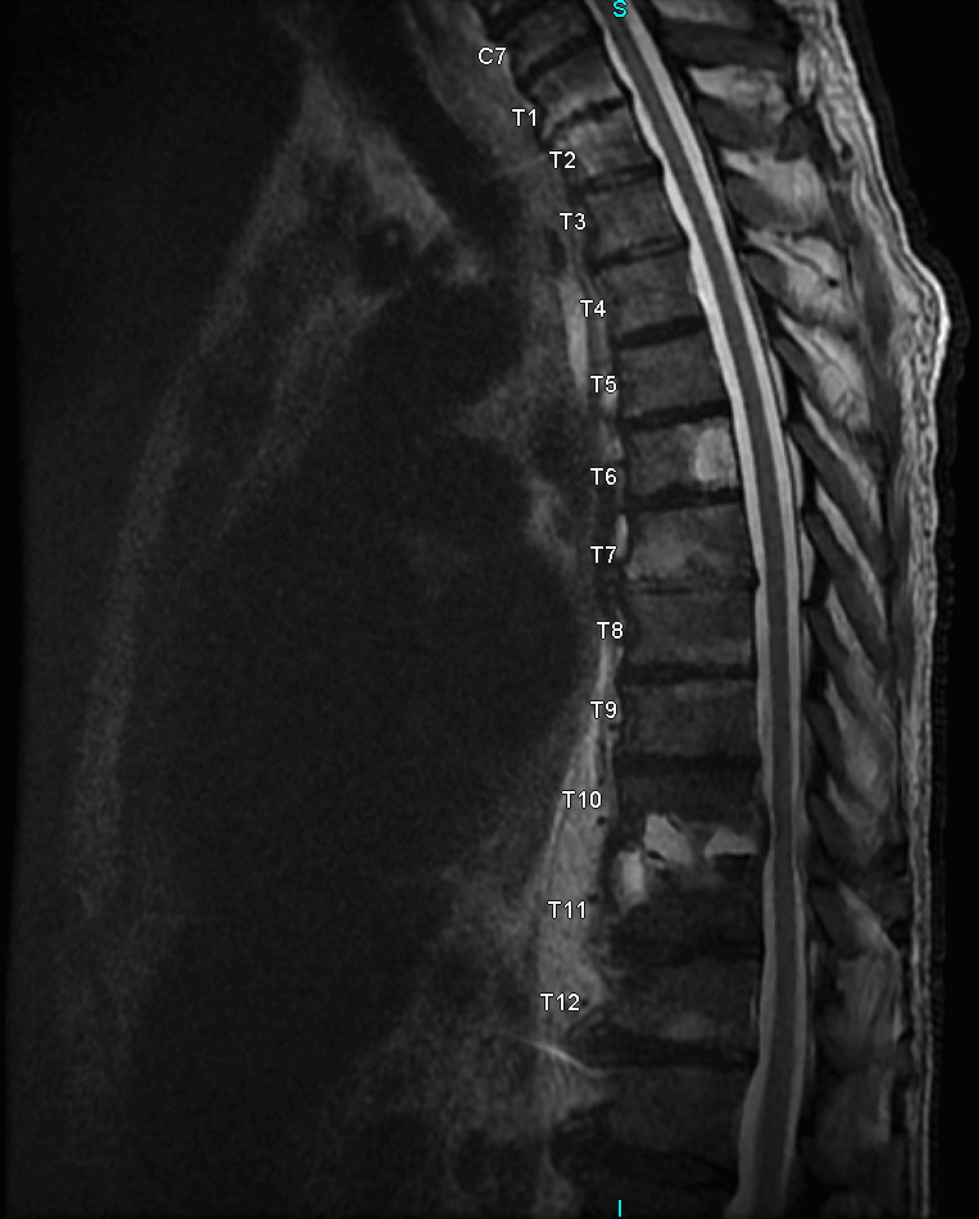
MRI of the thoracic spine without contrast Advanced vertebral body osteomyelitis and discitis at T10-11 with significant adjacent edema and inflammation. There is trace extension into the subligamentous spinal canal, including a posterior disc bulge and partial effacement of the thecal sac. A gadolinium enhancement is visible on T6 displaying a large hemangioma that is not clinically significant.
